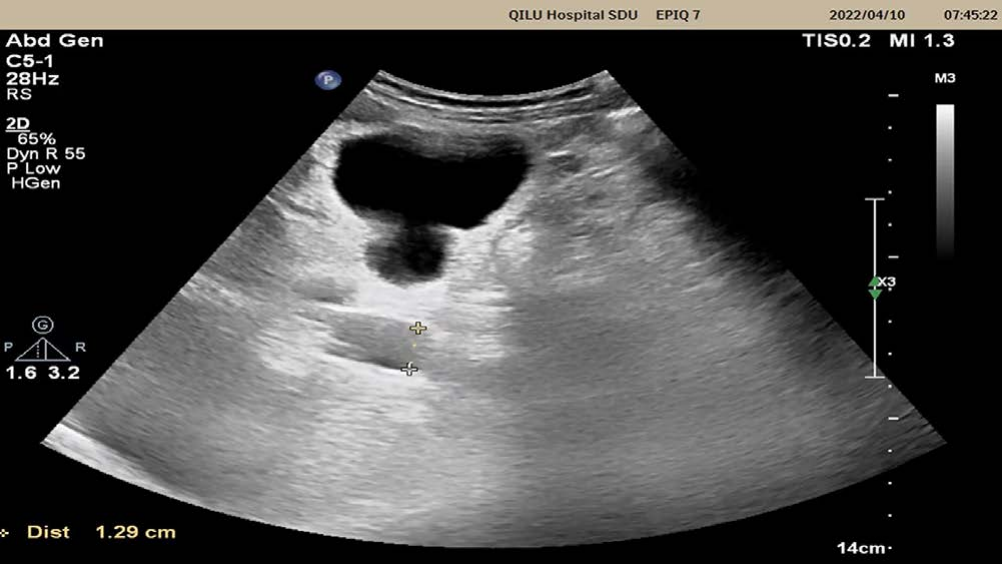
Ultrasound depicted a 13 mm dilated portal vein.
